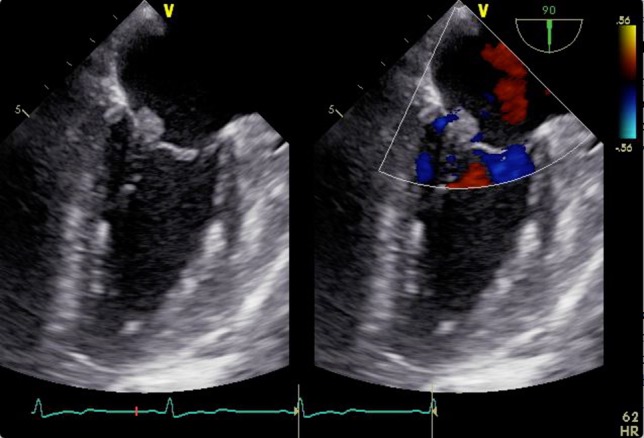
2D transesophageal echocardiogram showing a thickened mitral valve with vegetation that can be seen on the anterior mitral valve leaflet. Image on the right is a color flow Doppler view showing no mitral regurgitation and stenosis.
